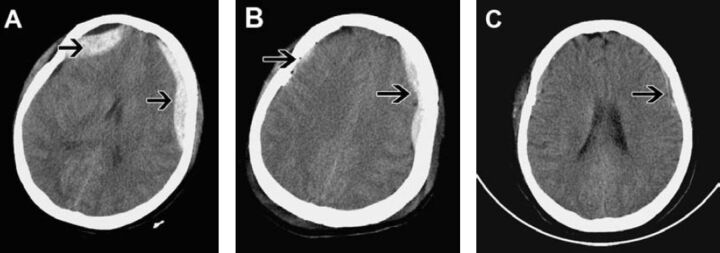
A 47-year-old female underwent single-site surgery, with the remaining hematoma treated non-surgically. Non-contrast axial head CT scans showing: A) pre-operative left fronto-temporal subdural hematoma and right frontal epidural hematoma; B) post-operative scan; C) follow-up, good recovery.
